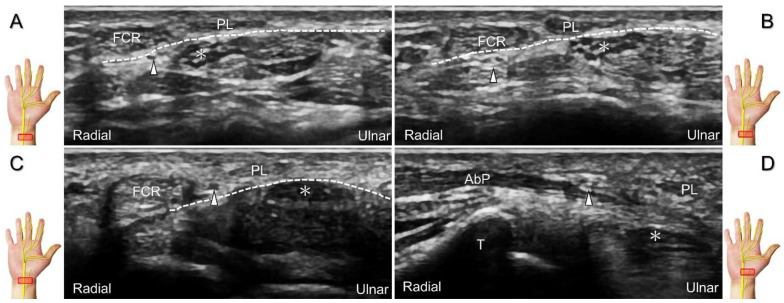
Sonographic imaging of the palmar cutaneous branch of the median nerve (A) shows its emerging from the radial aspect of the median nerve (B), penetrating the antebrachial fascia (C), and arriving at the superficial site of the abductor pollicis brevis muscle (D). Asterisk: median nerve; arrowhead: palmar cutaneous branch of the median nerve; dashed line, antebrachial fascia; FCR: flexor carpi radialis tendon; T: trapezium; PL: palmaris longus tendon; AbP: abductor pollicis brevis muscle.
